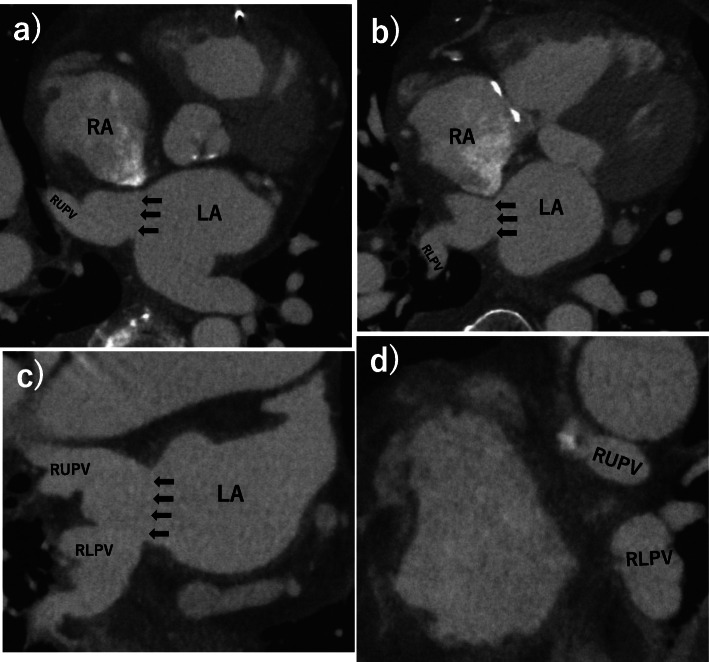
Postoperative multidetector computed tomography angiography. Postoperative multidetector computed tomography angiography demonstrating the intra-atrial baffle that show neither the pulmonary vein stenosis nor vena caval obstruction. Surgically created atrial septal defect in an appropriate size (black arrow) was recognized. Axial (a, b), coronal oblique (c), and sagittal oblique (d) images are presented. LA = left atrium, RA = right atrium, RUPV = right upper pulmonary vein, RLPV = right lower pulmonary vein
